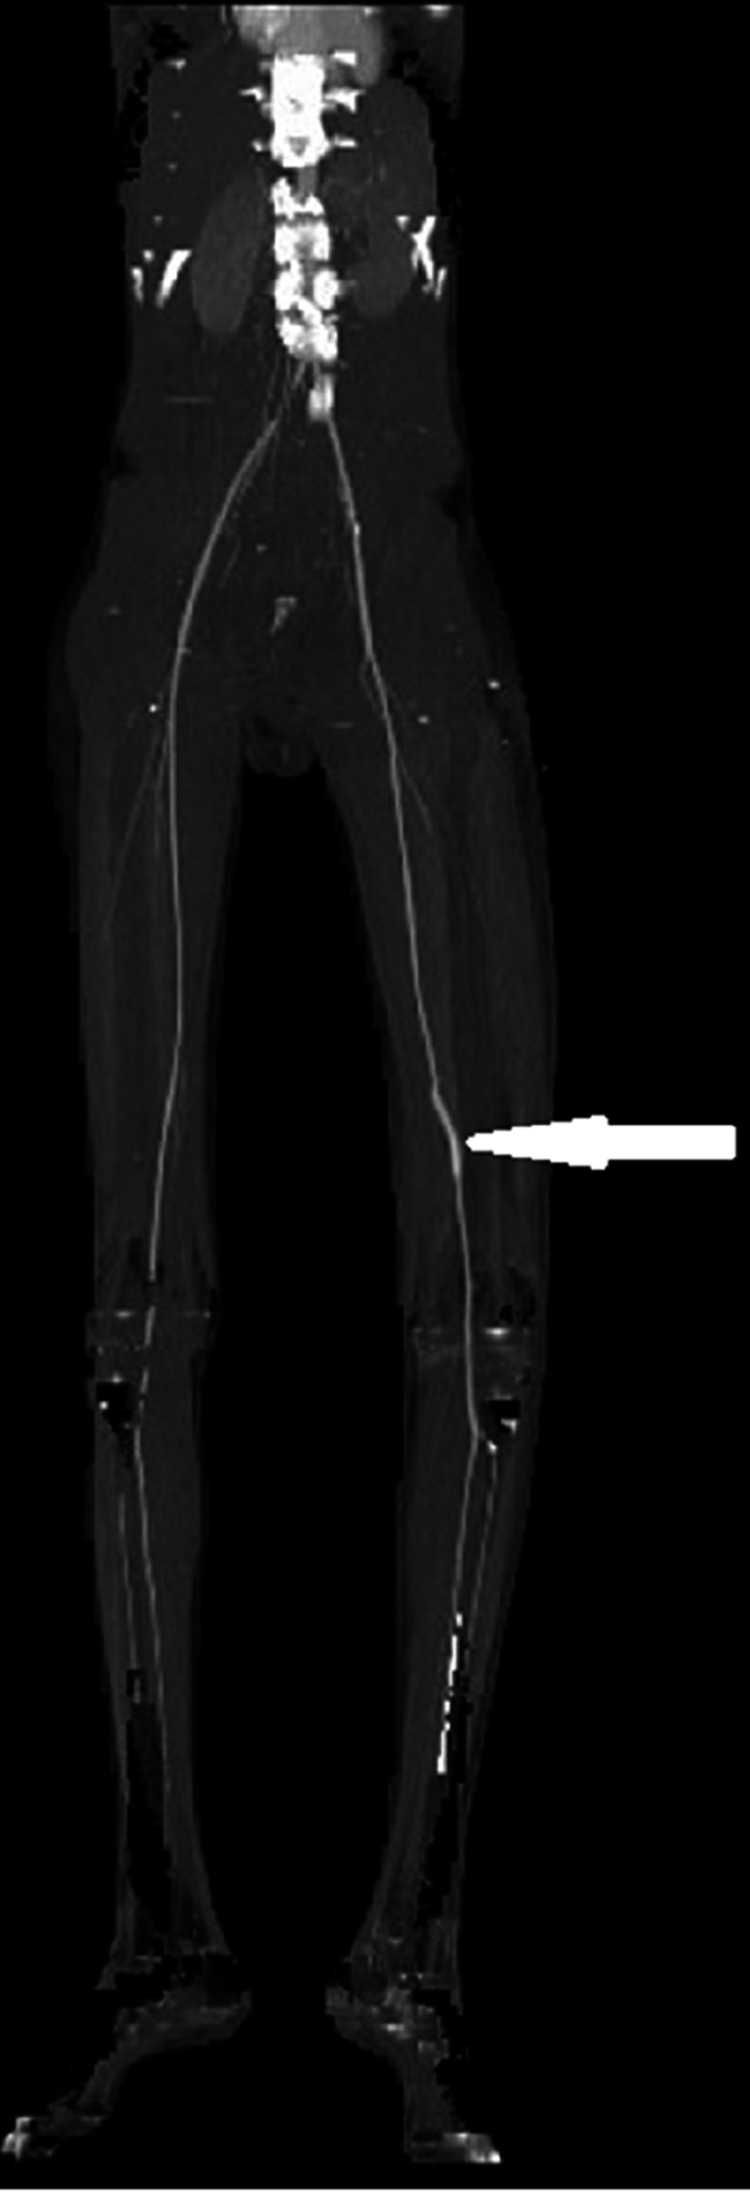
2D reconstruction of a CTA scan control performed one month postoperatively and showing a functional venous bypass (white arrow)
